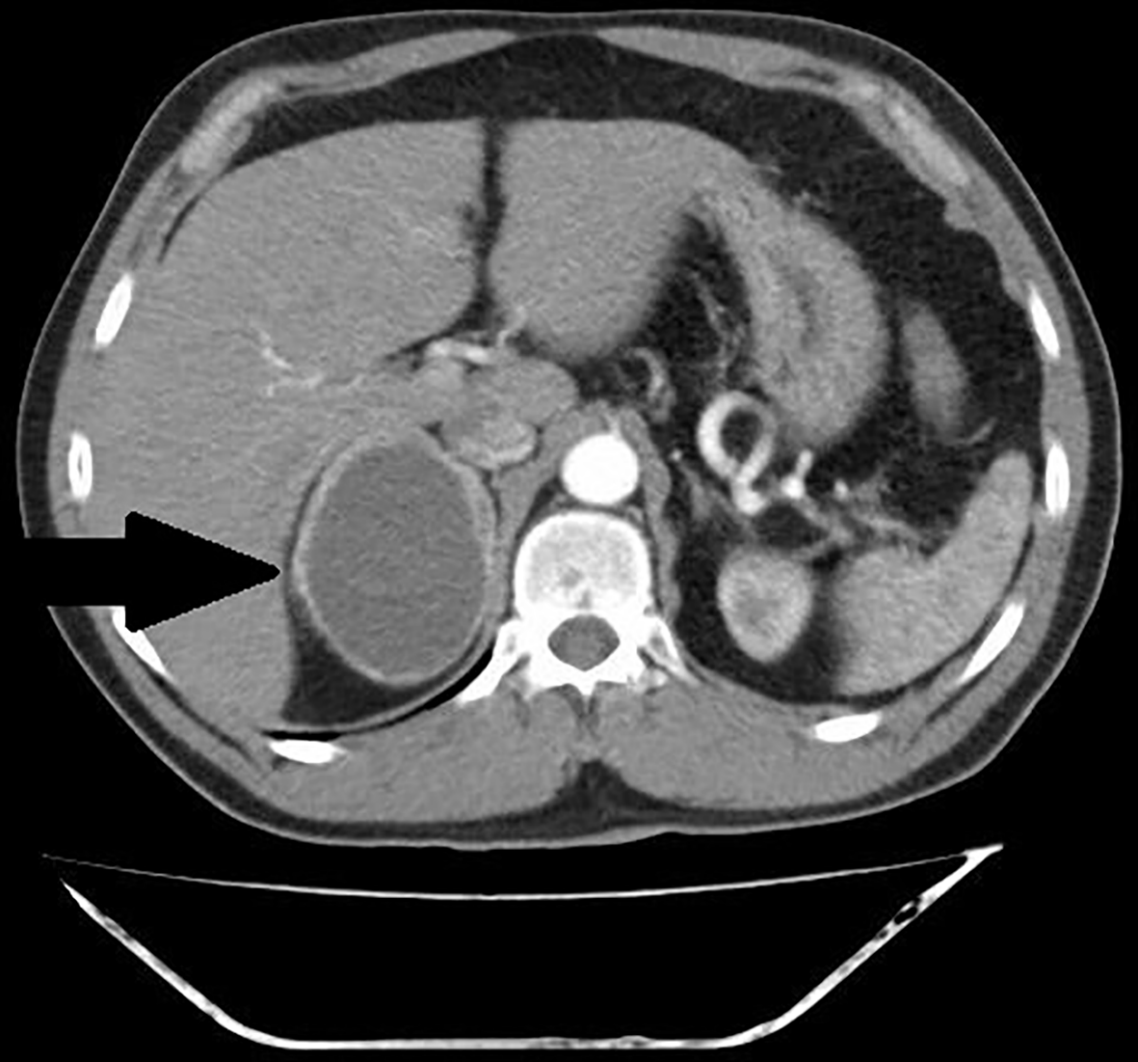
Patient number 4: CT of the abdomen, arterial phase, axial image- thick – walled hemorrhagic cyst of right adrenal gland with strong capsule-contrast enhancement (thick arrow).
